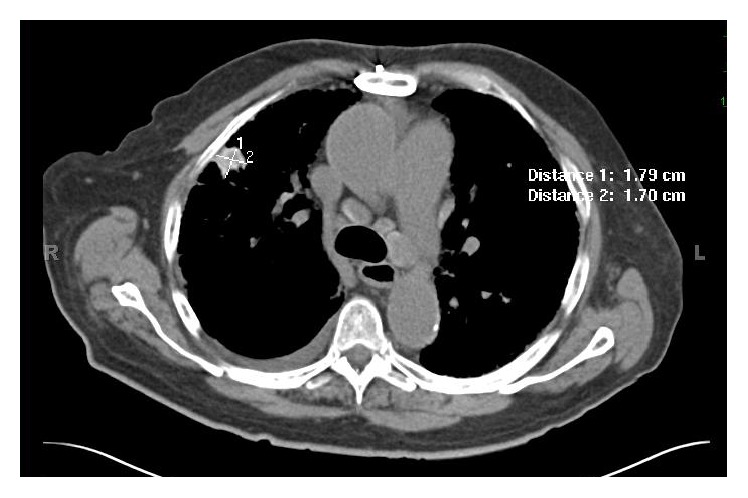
HRCT showing no interval change, when compared to CT angiogram, of multiple right upper lobe pulmonary parenchymal nodules, right pleural effusion, and diffuse fibrotic changes. The most prominent nodule measures 1.8 × 1.7 cm.
